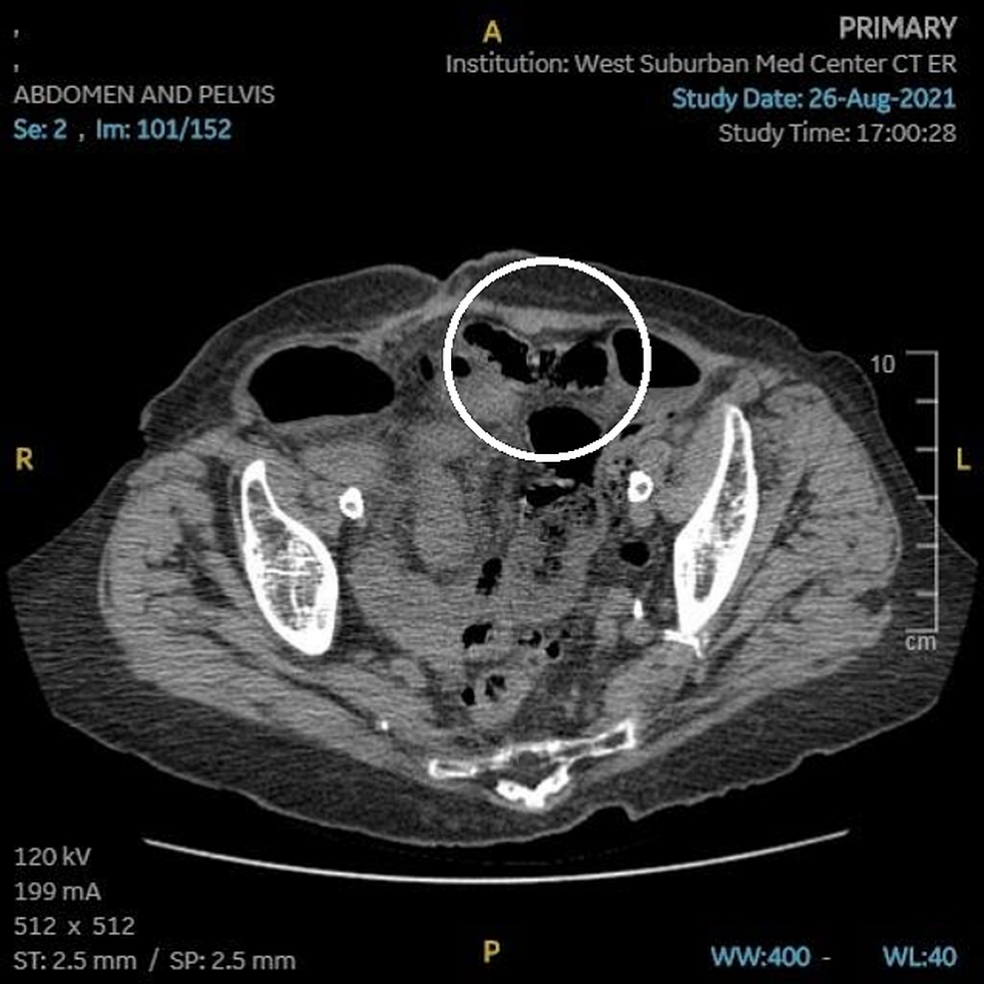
Computed tomography (CT) obtained prior to the first exploratory laparotomy, showing a segment of abnormal-appearing small bowel in the pelvis with wall thickening (circled).
